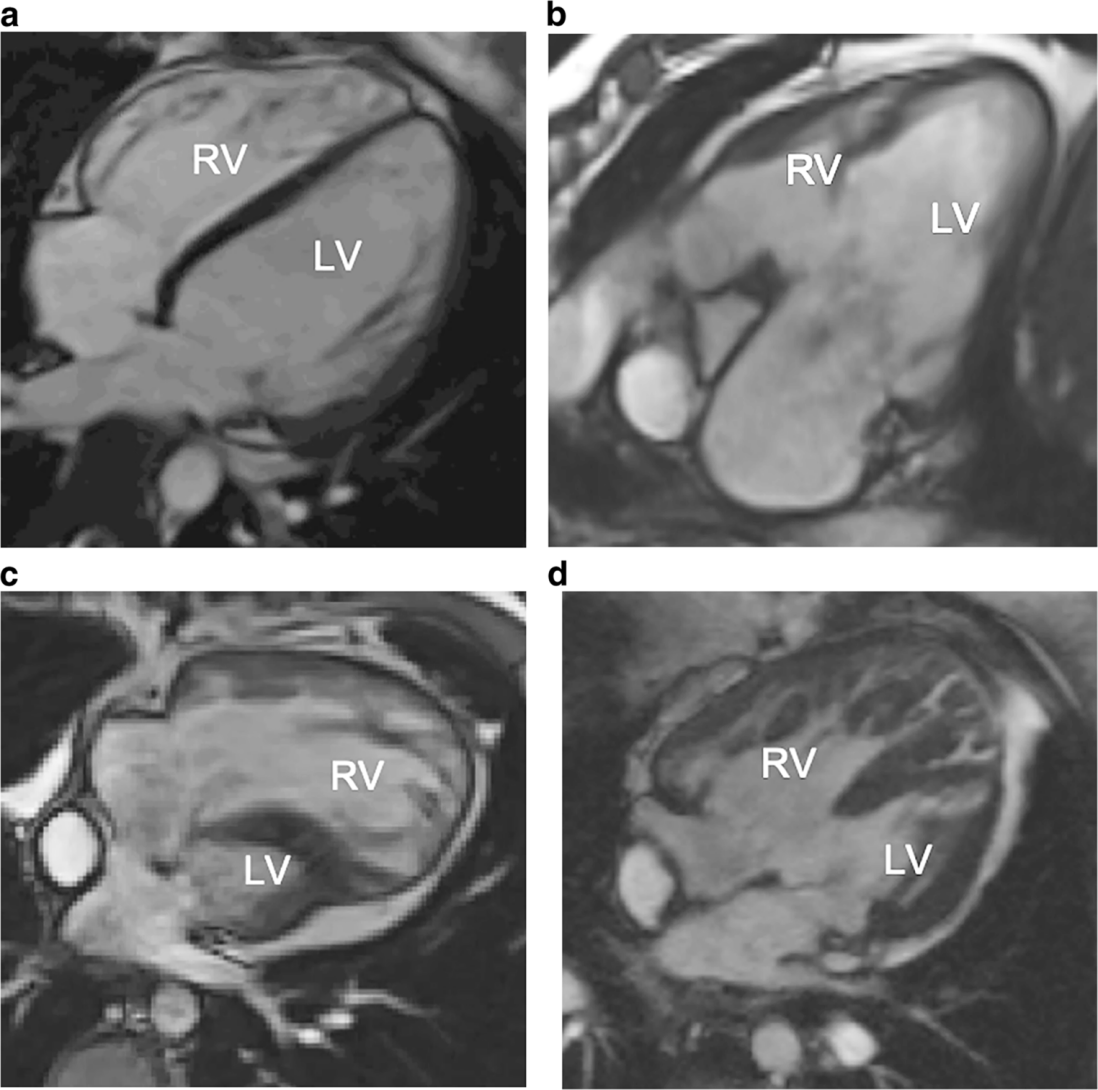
Cardiac anomalies with indication for Fontan procedure. a–d Cardiac MRI, 2-D steady-state free precession sequence, four-chamber view in (a) a normal 17-year-old boy, (b) a 16-year-old girl with tricuspid atresia with a small right ventricle, (c) a 15-year-old boy with hypoplastic left heart syndrome with a small left ventricle and (d) a 14-year-old girl with double-outlet right ventricle with a large ventricular septal defect with a slightly smaller right ventricle. *LV* left ventricle, *RV* right ventricle
